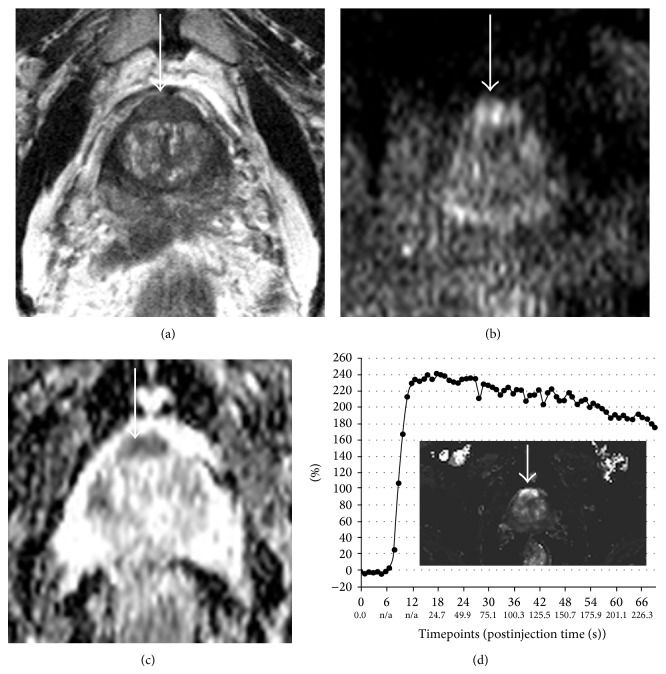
Multiparametric magnetic resonance imaging (mpMRI) detects significant prostate cancer. This 58-year-old man had a doubling of serum PSA in less than 2 years. For image-fusion targeted biopsy planning, mpMRI was performed. An oval, uniformly low signal mass (thick arrow) on T2-weighted imaging (T2WI) with circumscribed margins (a) is associated with focal high signal on diffusion-weighted imaging (DWI) (b) and low signal on the apparent diffusion coefficient (ADC) map (c) signifying restricted diffusion and focal asymmetric enhancement with washout (d) on dynamic contrast-enhanced (DCE) perfusion imaging. Targeted biopsies of this area revealed high volume Gleason 4 + 3 = 7 cancer. Standard systematic or “blind” sextant biopsies, which normally preferentially target the posterior gland, were all negative for any cancer.
